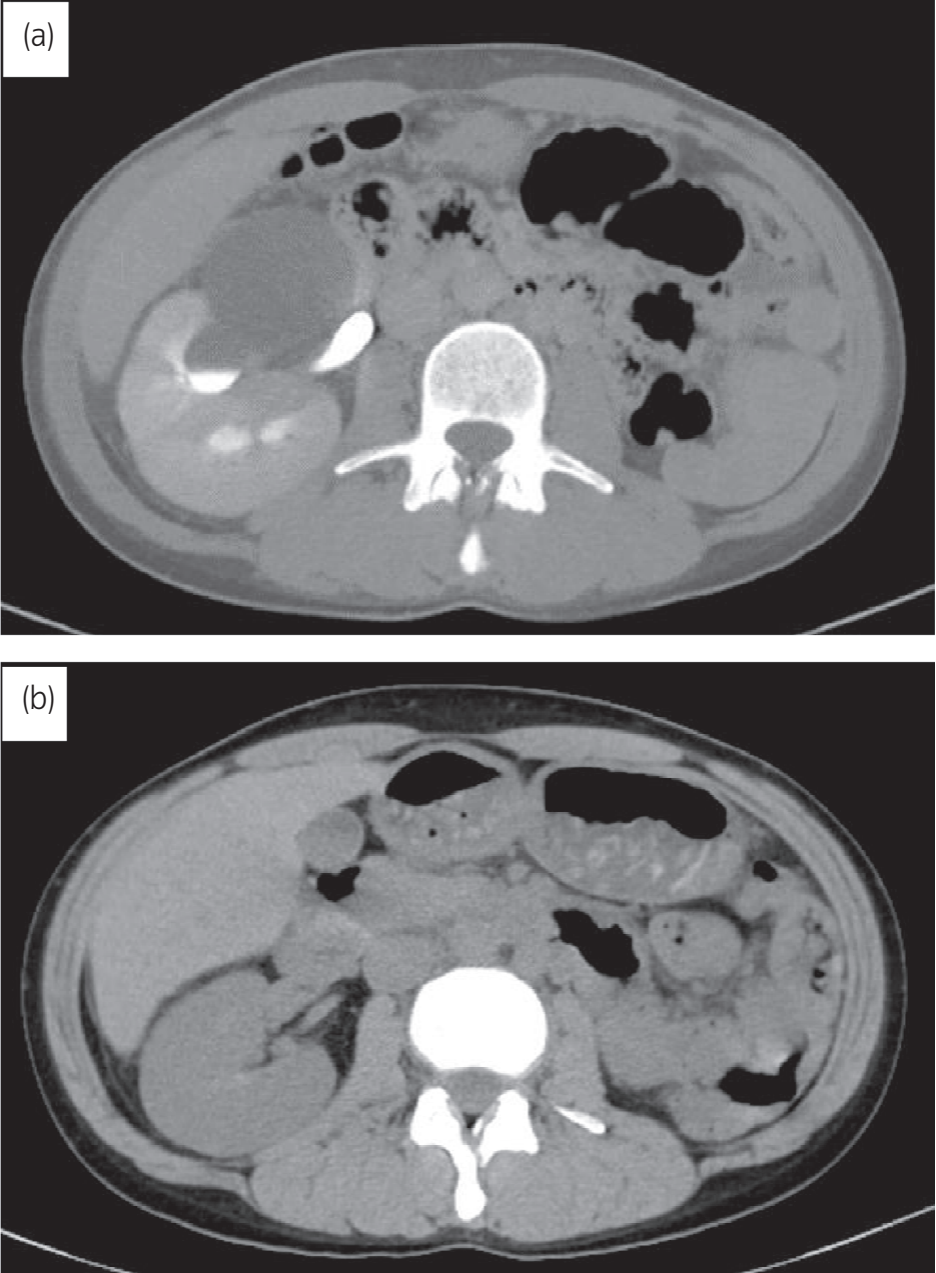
(a) CT urography showing hydronephrosis of lower pole of incomplete urinary duplication before pyeloureterostomy. (b) CT urography showing no hydronephrosis of lower pole of incomplete urinary duplication after pyeloureterostomy.
